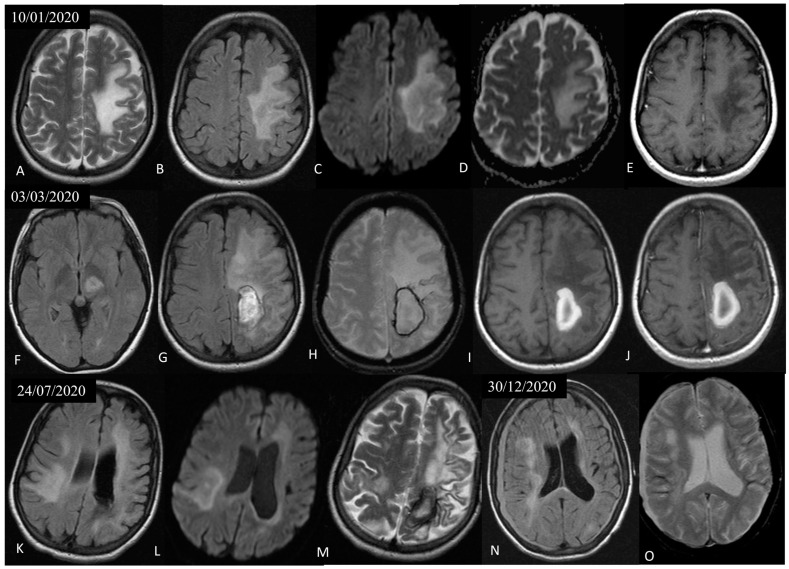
Axial T2 (A), FLAIR (B), DWI (C), ADC map (D), and post-Gadolinium T1 (E) sections of a follow-up brain MRI that was performed 1,5 months after the initial MRI, showed expansion of the pre-existing non-enhancing, T2 hyperintense, white matter lesion in the left frontoparietal area, with characteristic involvement of the U-fibers, lack of mass effect, and peripheral restricted diffusion, indicative of PML. Two months later, axial FLAIR (F,G), GRE (H), and pre- (I) and post-Gadolinium T1 (J) sections of a follow-up MRI showed further progression of the non-enhancing left frontoparietal lesion, a new lesion in the left thalamus, extending to the mesencephalon, and a subacute left parietal hematoma. Two more follow-up MRIs 5 months (axial FLAIR (K,L) and T2 (M)) and 10 months (axial FLAIR (N) and GRE (O)) later revealed new lesions in the right frontotemporal and parietal areas, with imaging characteristics also suggestive of PML, further loss of brain parenchyma and cortical hemorrhagic deposition in the left hemisphere, as well as bilateral frontoparietal subdural hematomas.
